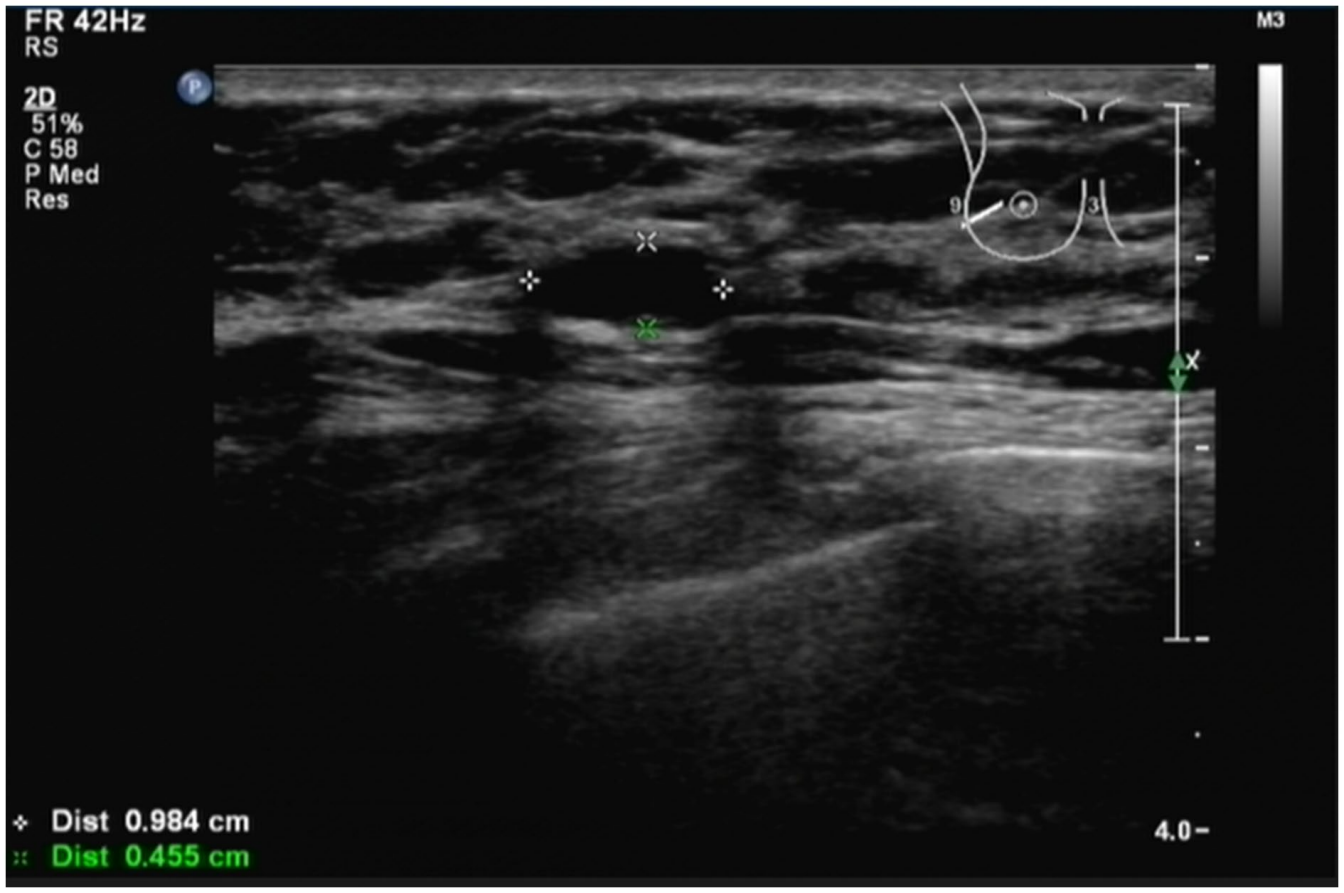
Patient was diagnosed with breast cancer by B-ultrasound. B-ultrasound examination showed that the size of the tumor in the left breast was 0.984 cm*0.455 cm. The patient had a history of breast cancer for 2 years.
